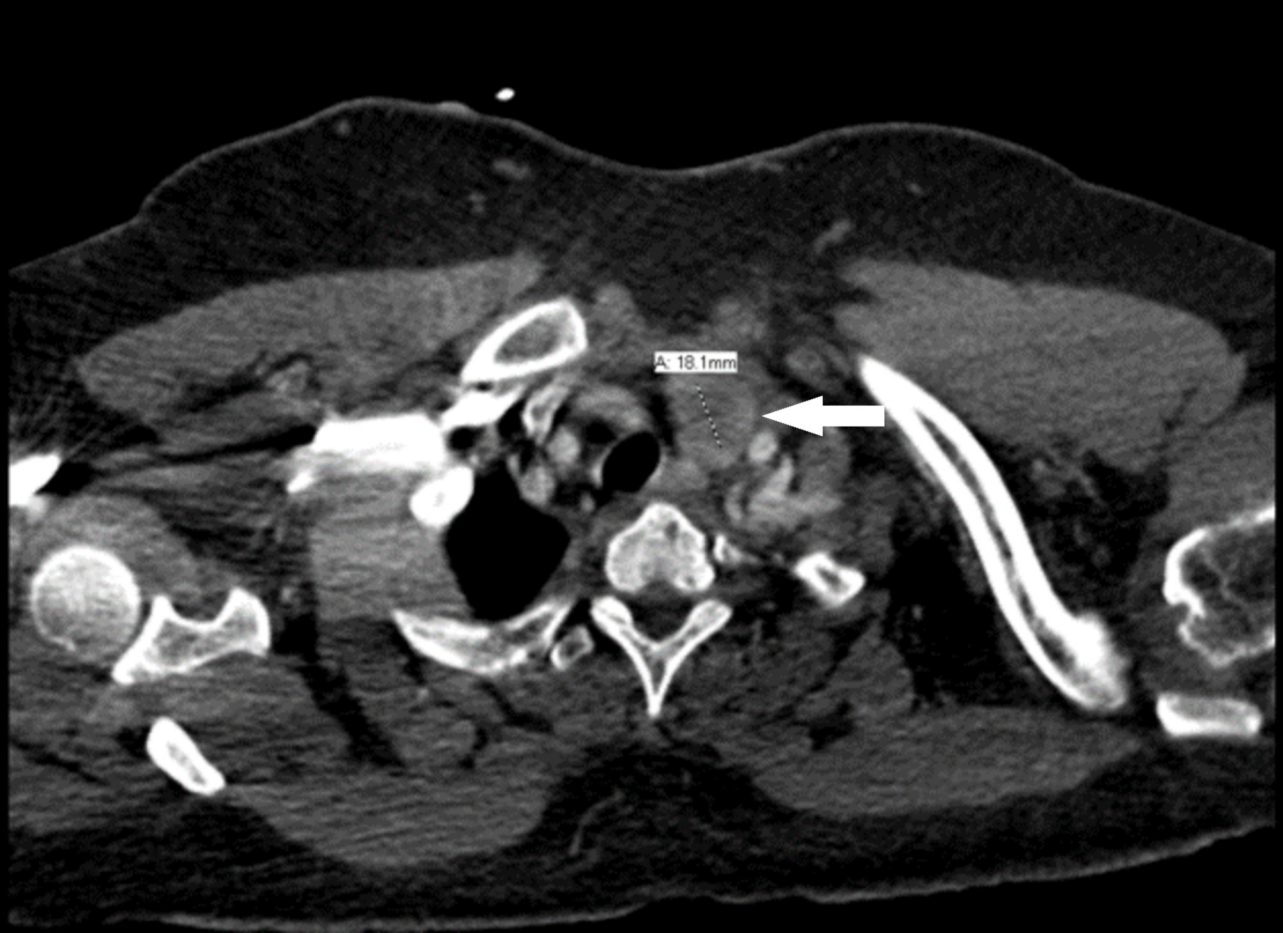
Computed tomography angiogram of the chest showing thyroid nodule
